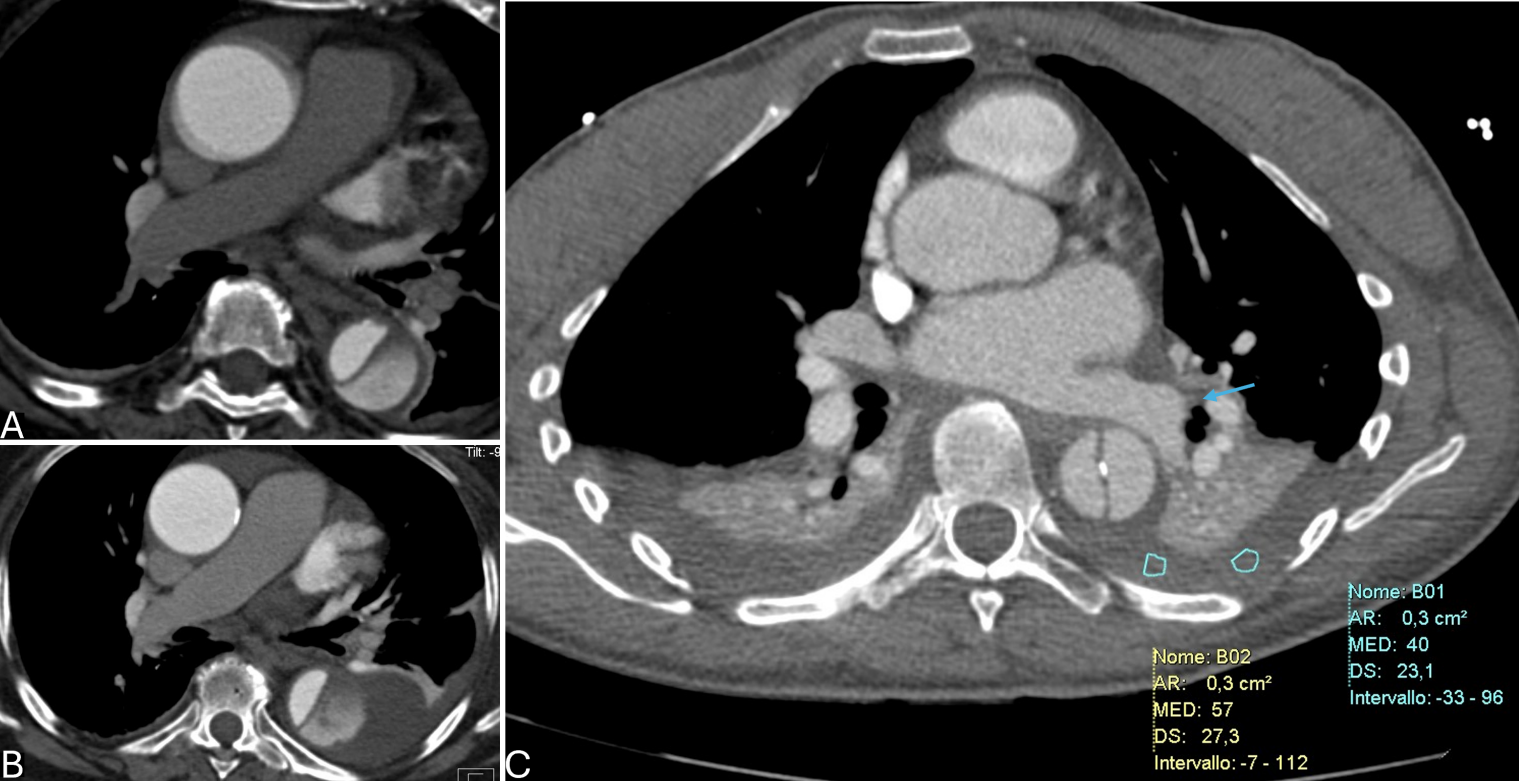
Complicated type B aortic dissection (TBAD) at computed tomography angiography (CTA): impending rupture signs. (A,B) Rapidly increasing
